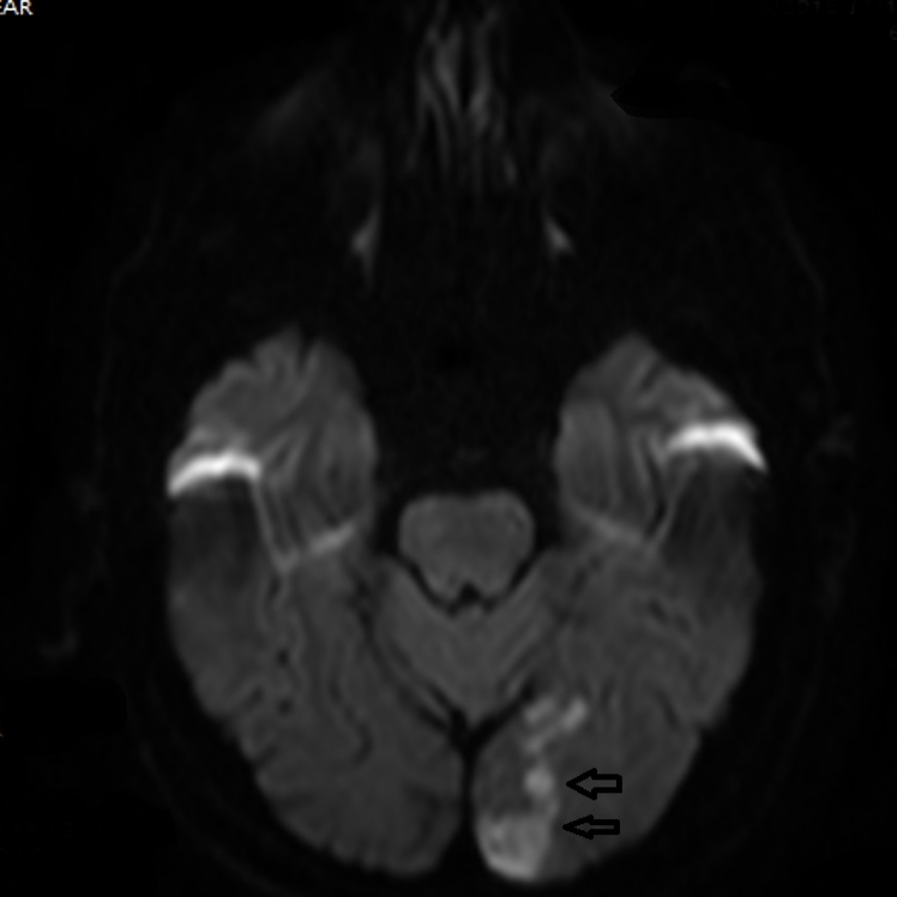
Magnetic Resonance Imaging of Brain Arrows point toward the area of infarct.
